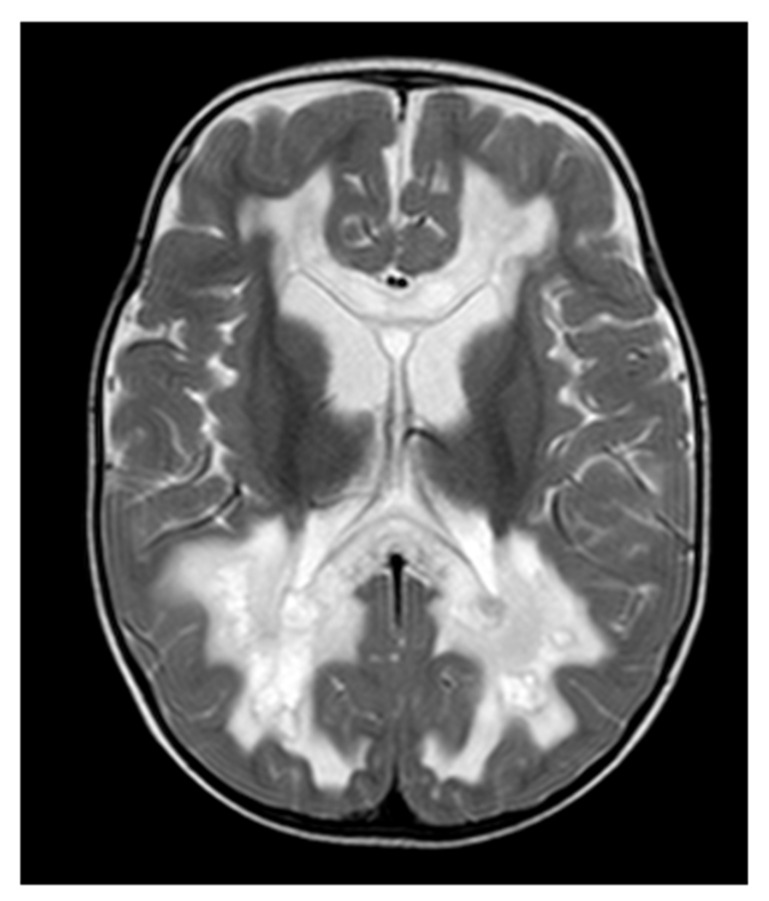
Neuroimaging in PDSS1 deficiency: Brain MRI (T2-weighted, axial view) of a 24-month-old boy with PSSD1 deficiency. Extensive leukoencephalopathy is visible with cystic white matter lesions in the occipital regions. Other MRI images of this individual were published previously [16].
